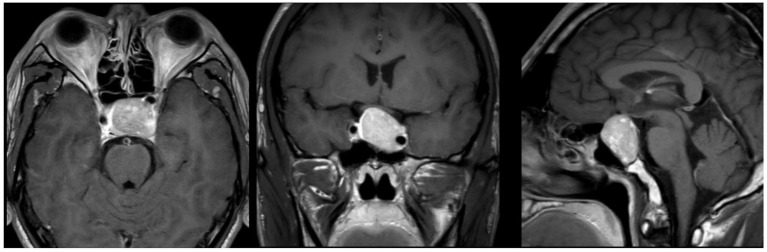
Cranial magnetic resonance imaging (MRI) reveals a pituitary tumor measuring 2.9 × 2.6 × 3.3 cm.
